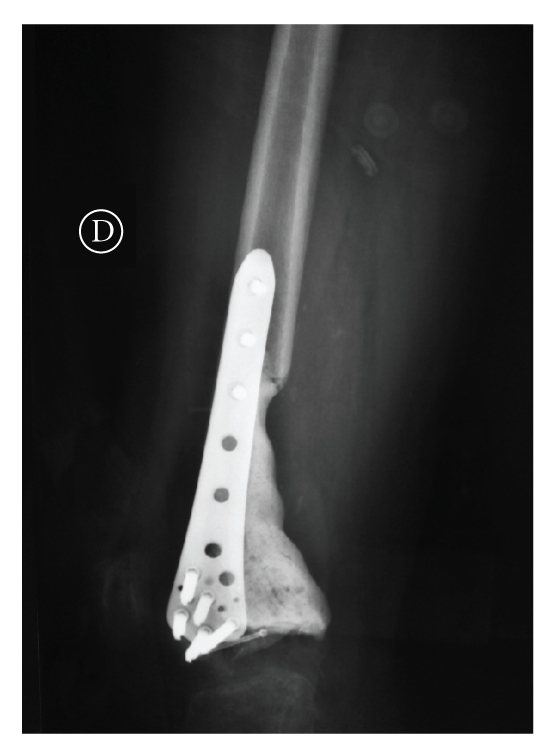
Lateral radiography of the reconstructive technique at the one-year follow-up.
